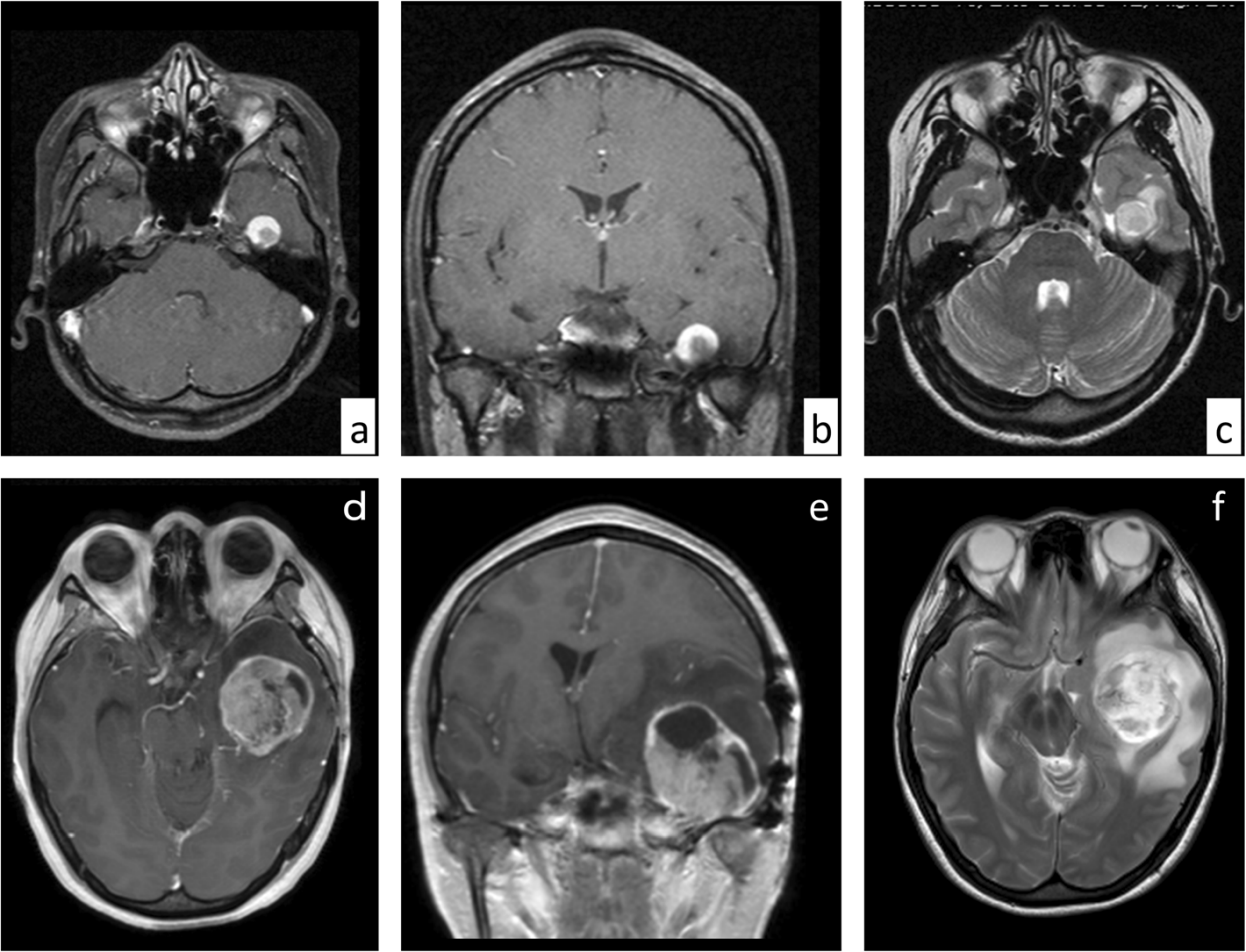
Neuroimaging along the course of the disease. T1-weighted MR images with gadolinium contrast (a, b) revealing a well-defined, heterogeneously enhanced tumor in the left temporal lobe, approximately 10 mm in size, with high intensity on T2-weighted imaging (c). Axial(d) and coronal(e) MR images with gadolinium contrast and axial T2-weighted images (f) showing local recurrence in the surgical cavity 12 years after the initial treatment with a size of approximately 42 × 45 × 47 mm
